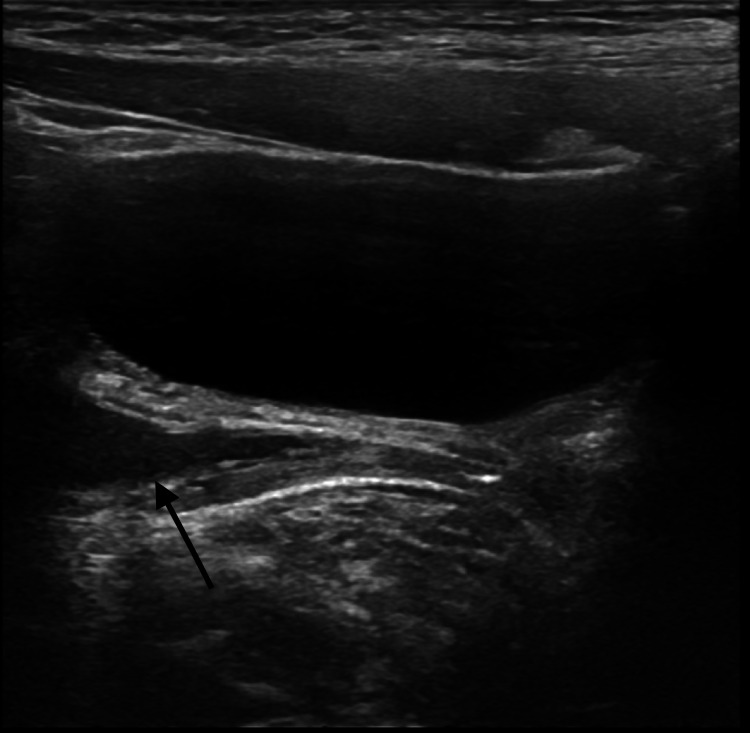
A US showing a well-defined elongated fluid-filled structure inserted into the proximal urethra (black arrow). US: ultrasound.
